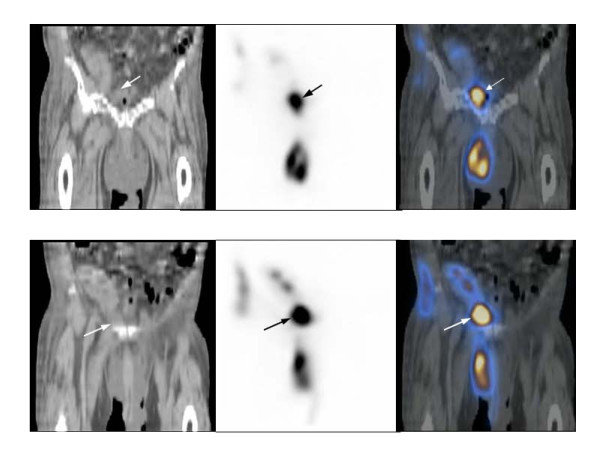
Coronal images of SPECT/CT of pelvis. (A) The images show a radiotracer accumulation demonstrating a urine leak (arrows) in the right pelvic cavity just next to the urinary bladder and in the scrotum. A Foley catheter balloon is located in the very contracted urinary bladder. (B) More anterior coronal slice shows a urine leak (arrows) in the right pelvic cavity just below the transplanted kidney extending to the scrotum and the right abdominal wall.
